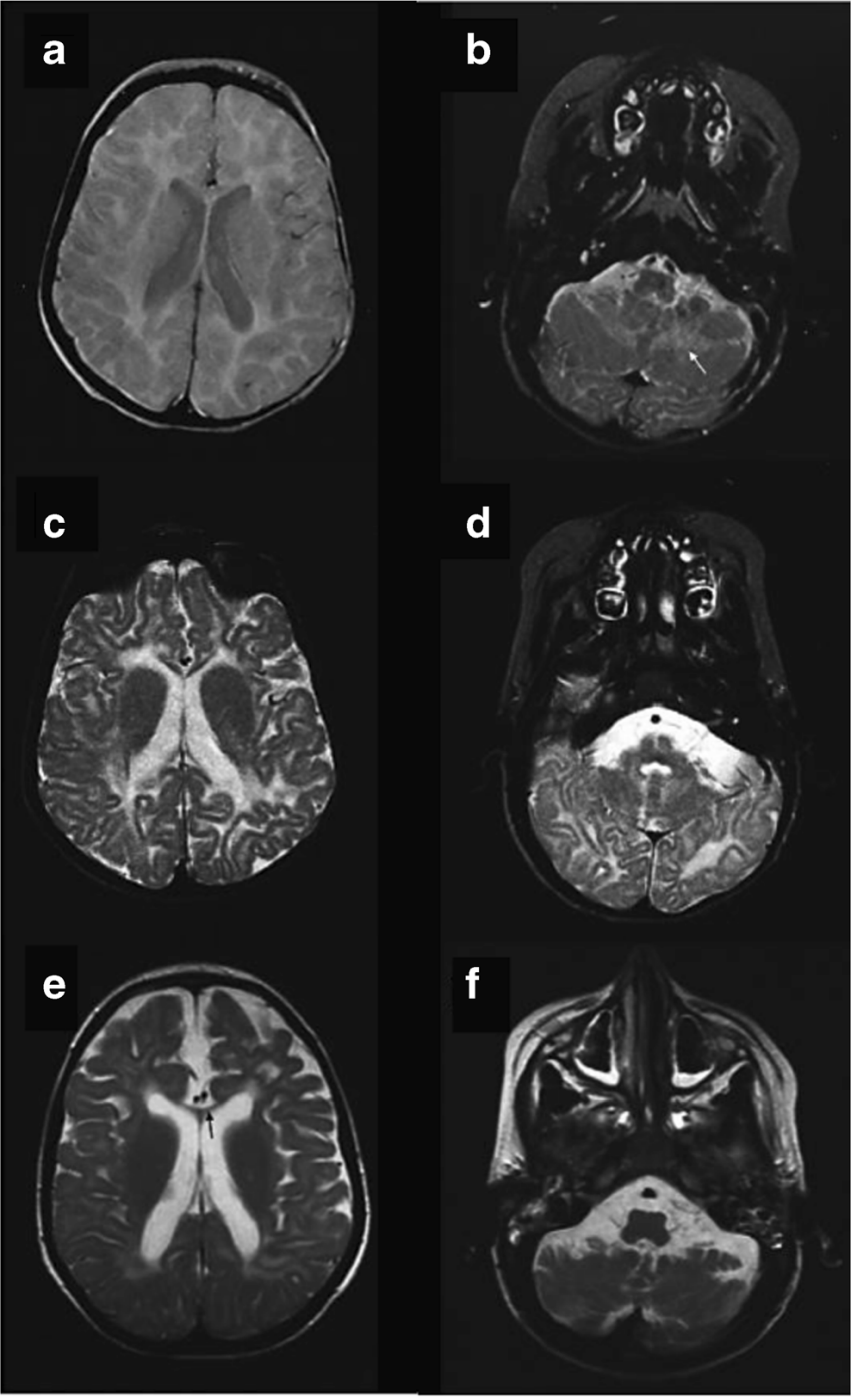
MR imaging of the brain in patient 1 at the age of 12 (a,b), 27 (c,d) and 73 months (e,f). Axial images at the level of the basal ganglia (a,c,e) as well as the cerebellum (b,d,f) show signal changes which indicate hypomyelination: there is high signal on the proton density (a) and T2w (c,e) images of the supratentorial white matter. Inner and outer CSF spaces were mildly enlarged on follow-up and corpus callosum got thin (*black arrow* in e) corresponding to atrophy. The cerebellum was not specifically affected by atrophy, but cerebellar white matter was also hypomyelinated, best seen at age 12 months (b), *white arrow*
